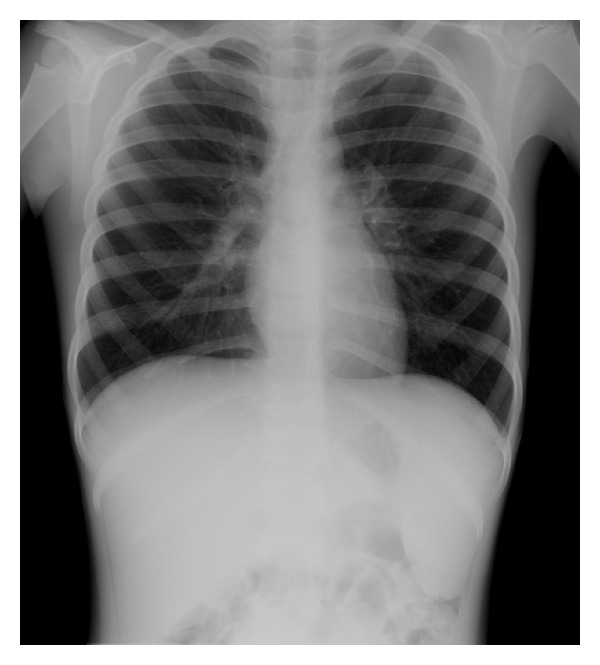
A chest radiograph of the patient showing infiltration in the lower right lung and mild splenomegaly.
